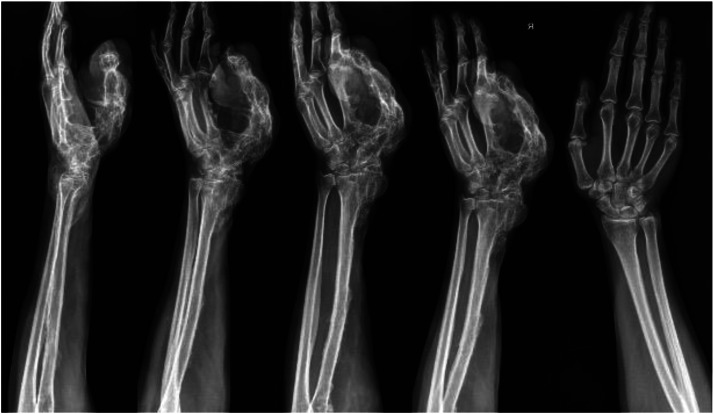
The patient's hand radiographs before surgical treatment.
